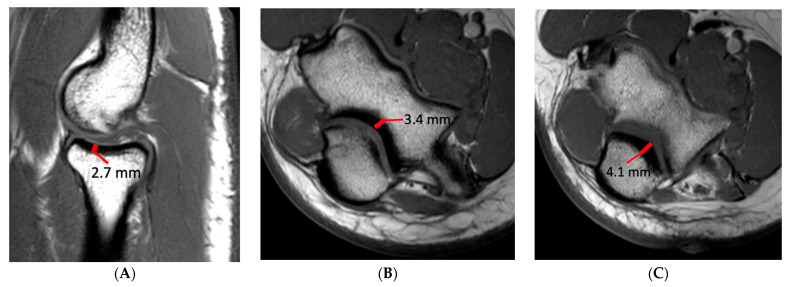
A 16-year-old male baseball pitcher with images demonstrating the measurement technique for quantifying subchondral sclerosis (thick red line). (A) Sagittal T1-weighted image demonstrating the measurement technique at the radial head, taken parallel to the articular surface; (B) axial T1-weighted image demonstrating the measurement technique at the posterior humeral trochlea, again made parallel to the articular surface; (C) axial T1-weighted image demonstrating the measurement technique at the olecranon process.
